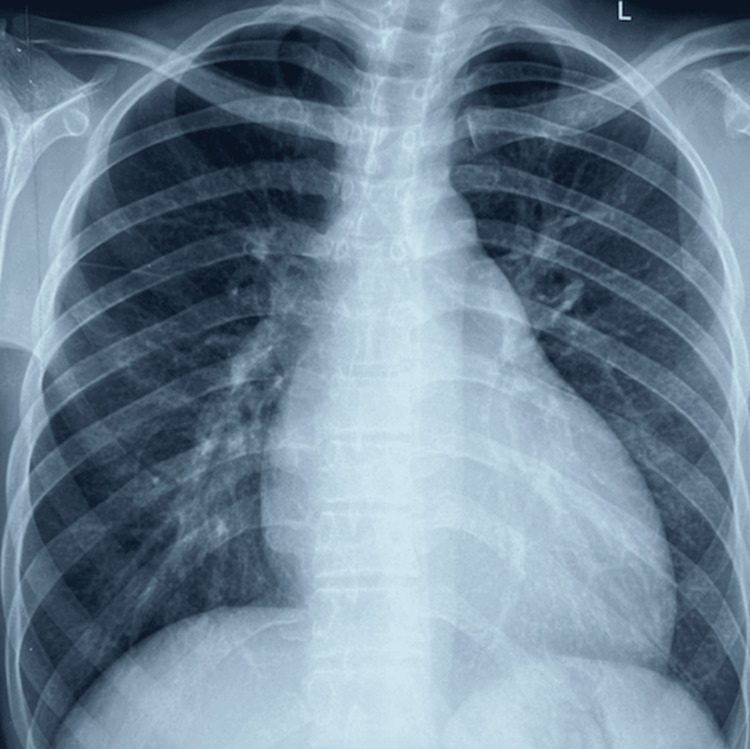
Chest X-ray with mild cardiomegaly
